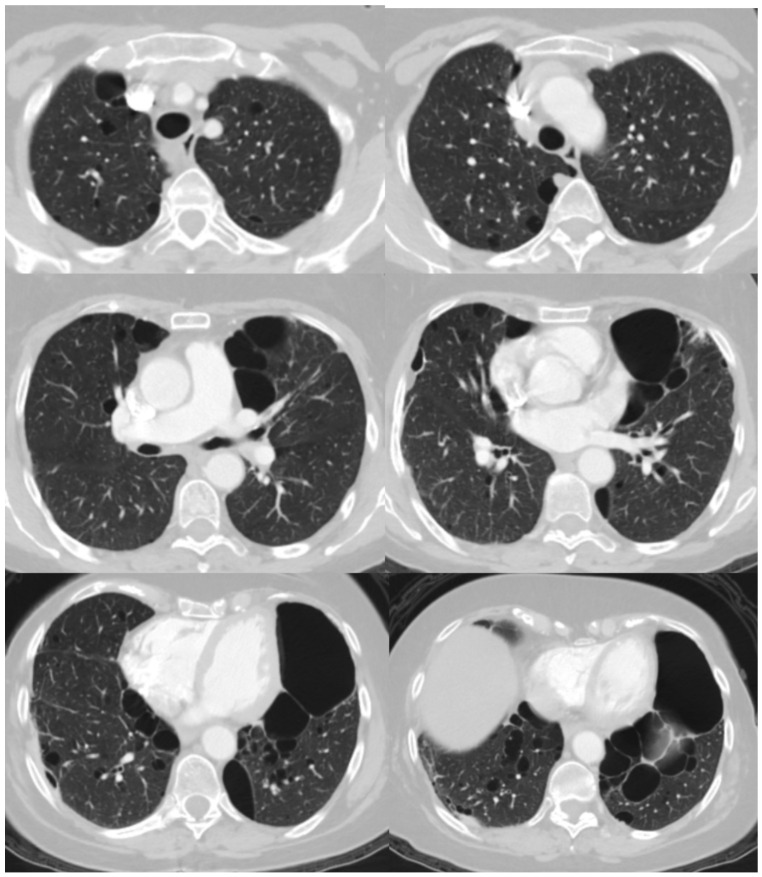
High-resolution chest computed tomography of a patient with BHD syndrome showing multiple, thin-walled pulmonary cysts with lower lung lobe predominance.
